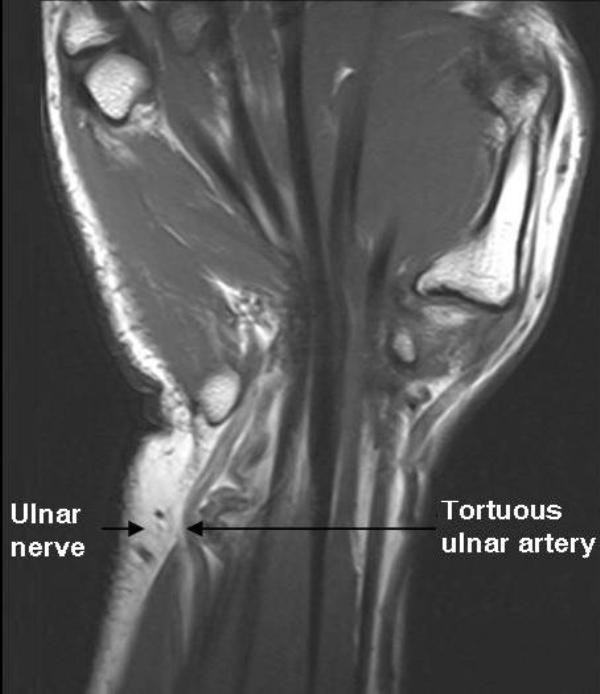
Coronal MRI image: Ulnar nerve compression in Guyon's canal due to tortuous ulnar artery.
